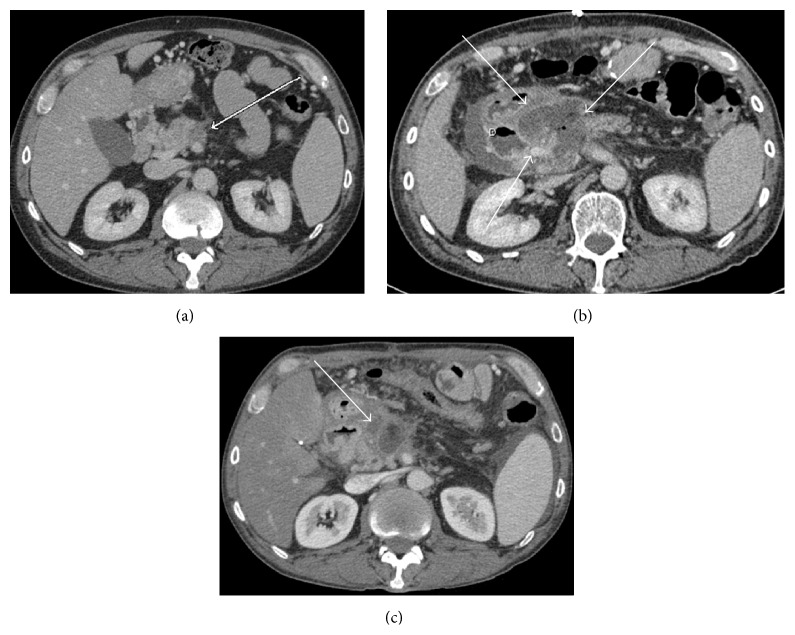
(a) Preoperative CT scan demonstrating soft tissue in the posterior aspect of the proximal pancreatic body (white arrow) extending posteriorly to surround at least 180 degrees of the proximal to midsuperior mesenteric artery. (b) Immediate postoperative CT scan demonstrating a large fluid collection in ablative bed with foci of internal gas. Ablative zone (white arrows) has been demarcated from nearby duodenum (D). (c) Three-month follow-up scan showing markedly decreased ablative zone with decreased size of the fluid collection (white arrow) consistent with no recurrent disease.
